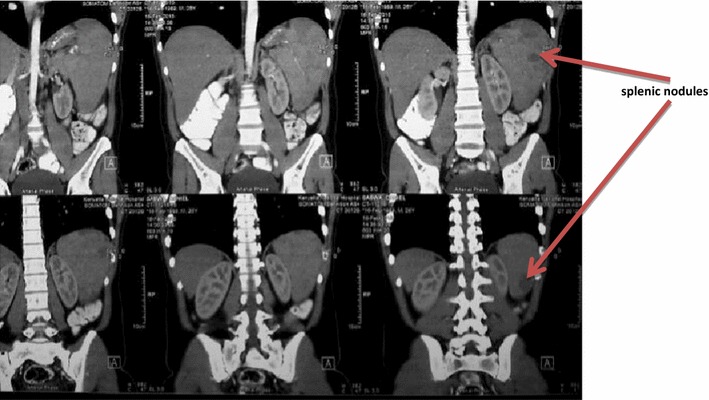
Computed tomography of the abdomen slices showing multiple splenic nodules
